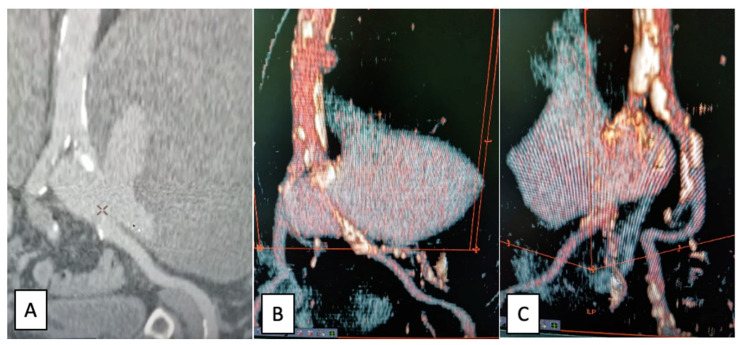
CT images of the left common iliac artery saccular aneurysm: (A)—coronal view; (B,C)—3D reconstruction.
